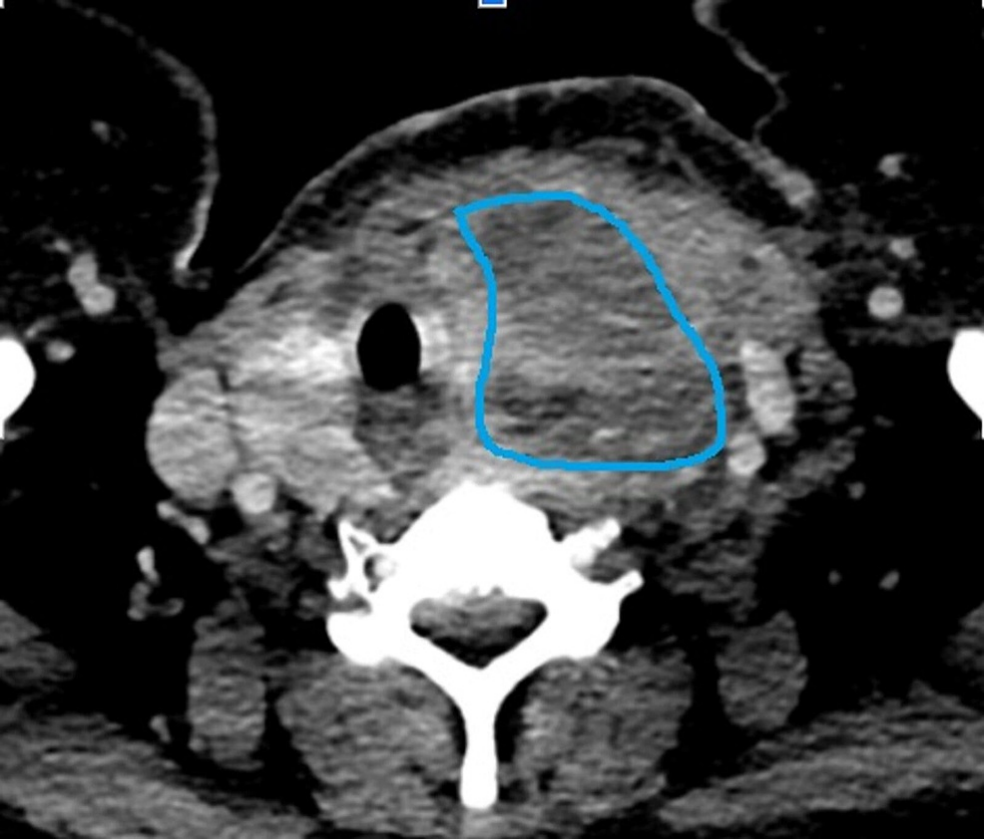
CT neck showed large thyroid mass with multiple hypodense area and fluid-filled content highly suggestive of necrosis or abscess (marked area). There was tracheal deviation.
